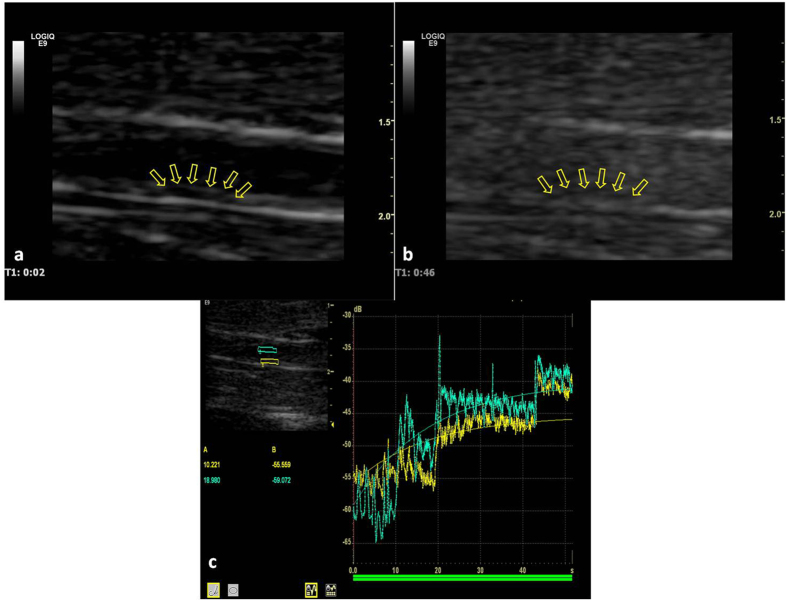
Contrast-enhanced ultrasound images of the abdominal aorta. (a) Longitudinal view obtained 2s after injection of contrast agent. No enhancement was observed in the plaque (arrows). (b) Longitudinal view obtained 46s after injection of contrast agent. Plaque (arrows) was enhanced after the injection of contrast agent. (c) The signal intensity-versus-time curve in the lumen of the abdominal aorta (blue area and curve) and plaque (yellow area and curve). A in panel c = enhanced intensity after injection of contrast agent; B in panel c = baseline intensity (background intensity) prior to the injection of contrast agent. When a plateau of the signal intensity-versus-time curve occurs, the derived peak signal intensity after injection of the contrast agent in the regions of interest and the baseline intensity (signal intensity at the origin of the curve) before injection of the contrast agent were obtained. The enhanced intensity was calculated by subtracting the baseline intensity from the derived peak signal intensity. The degree of enhancement of the plaque after injection of contrast material was investigated by measuring the enhanced intensity in the plaque and the ratio of the enhanced intensity in the plaque to that in the lumen of the abdominal aorta.
